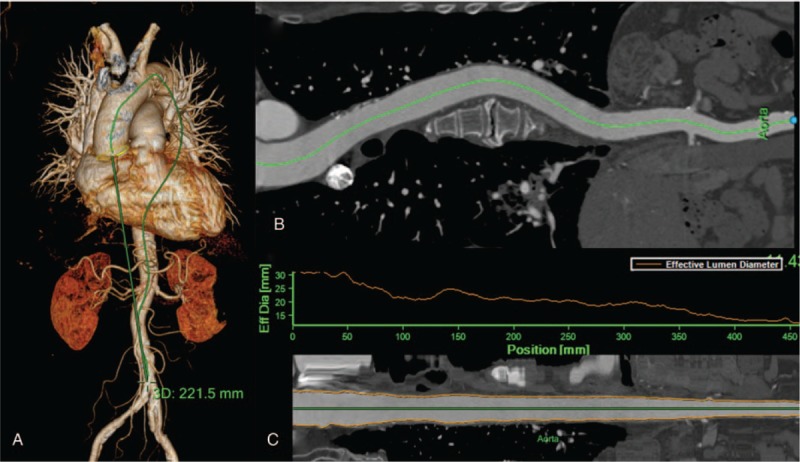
Measurement of aortic tortuosity. Three-dimensional volume rendering image of thoracoabdominal CT angiography shows that the distance between the aortic root and the aortic bifurcation is 221.5 mm. (A) Curved multiplarnar reconstruction image shows the centerline of the whole aortic lumen from the aortic root to the aortic bifurcation. (B) Straightened multiplanar reconstruction image shows that the length of the center line is 473.2 mm. Aortic tortuosity was estimated at 0.47 (= 221.5/473.2).
